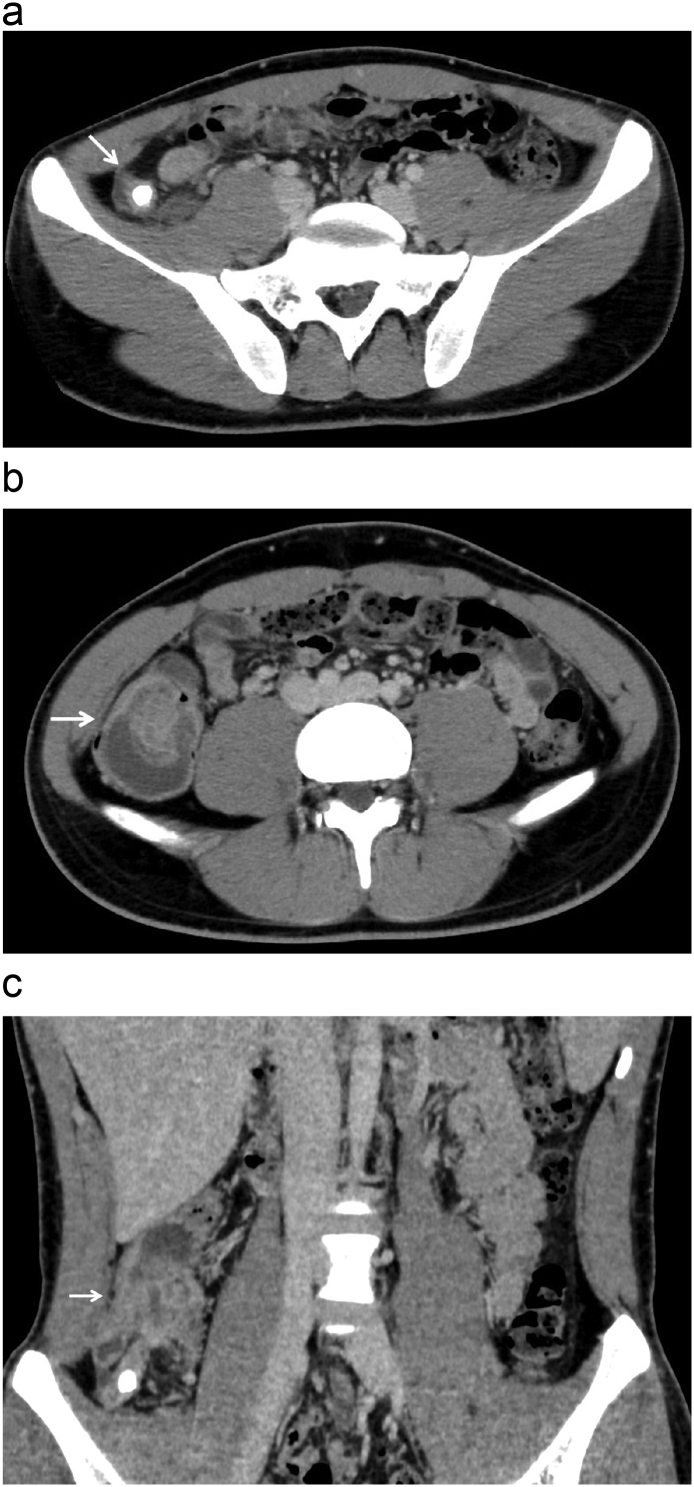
Computed tomography (CT) showed the swollen appendix and appendicolith in the middle of the appendix (a: axial plane) (white arrow). The target sign indicated that the cecum was intussuscepted to the ascending colon (b: axial plane) (white arrow). The colonic wall of the cecum and ascending colon showed edematous thickening (c: sagittal plane) (white arrow).
